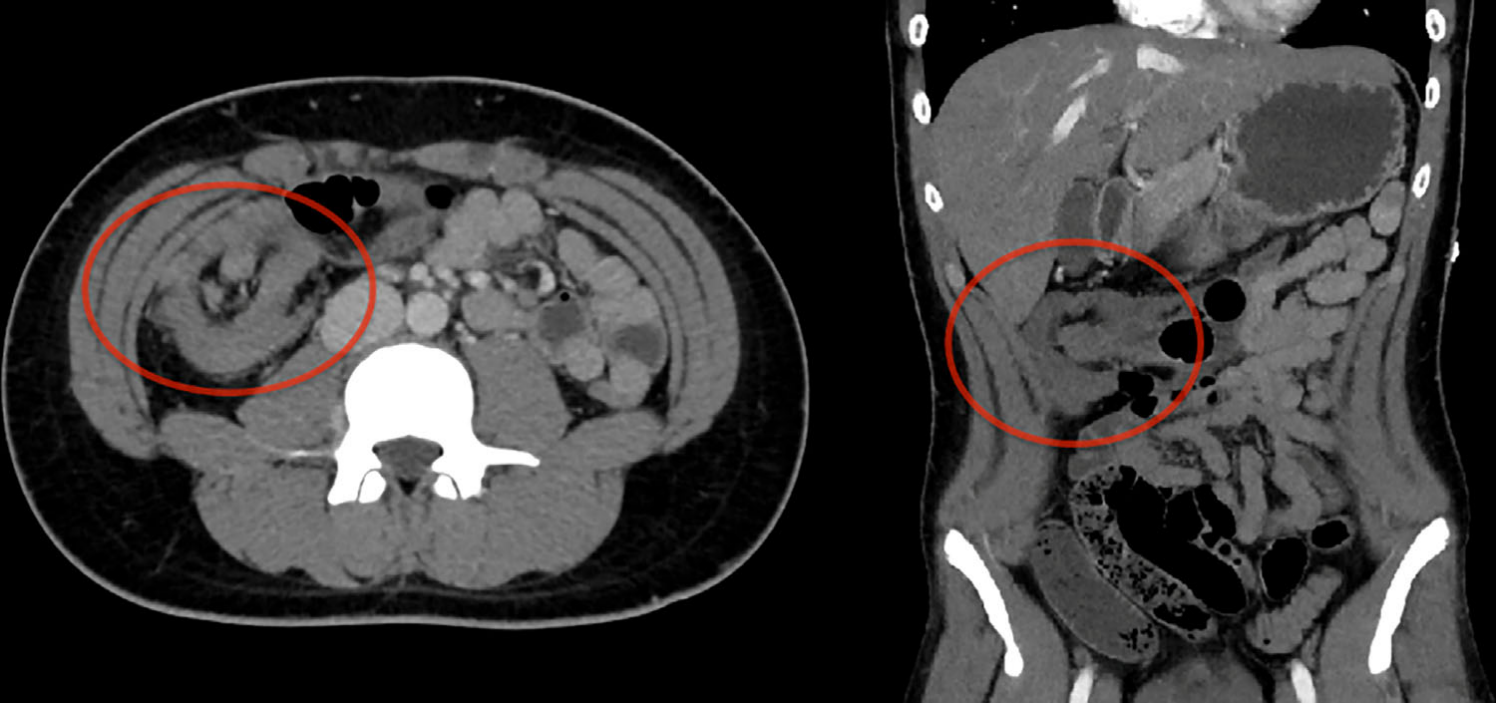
Computed tomography image on admission shows a target sign in the ileocecal region, indicating intussusception (red circle).
